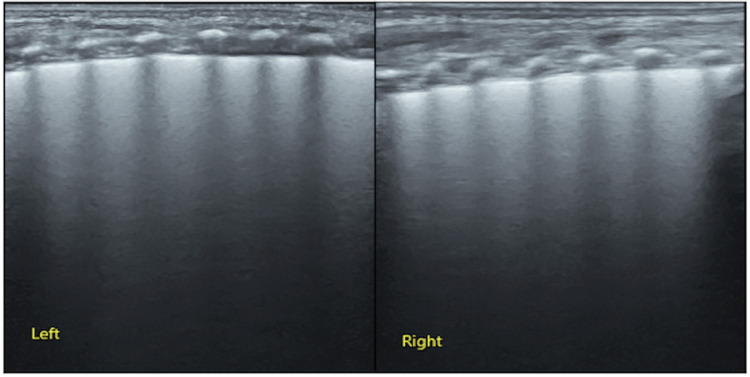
LUS examination results of Supplementary Video S2 (case 2). LUS showed confluent B-lines in both lungs suggesting significant lung edema, which is typical of ultrasound imaging of TTN. LUS, lung ultrasound; TTN, transient tachypnea of the newborn.
